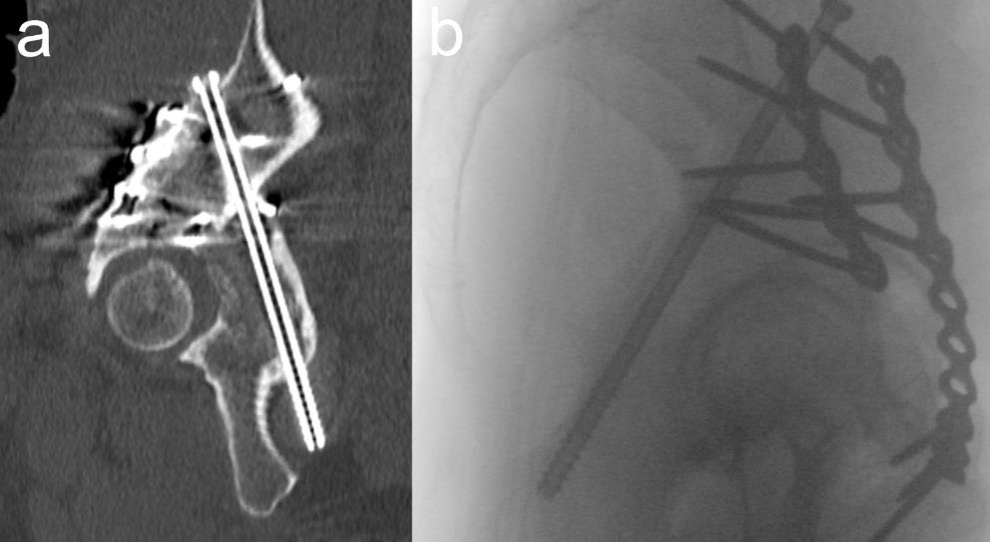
Cortical perforation of the posterior column. a Postoperative CT scan of an ACPHT fracture treated with PCS showing adequate reduction of the posterior hemitransverse fracture and no hip joint penetration, but posterior cortical perforation of the posterior column. Sagittal reconstruction. b The posterior cortical perforation is not clearly visible in a intraoperative lateral oblique view
